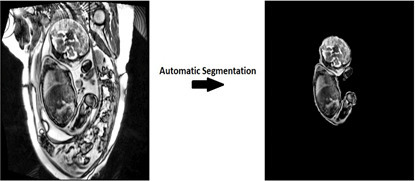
Example of how an automated segmentation algorithm (‘CASE-Net’) could be used to help extract only images of the fetal body from an intrauterine fetal MRI study. Reproduced from Lo J. et al^6^, 2021
